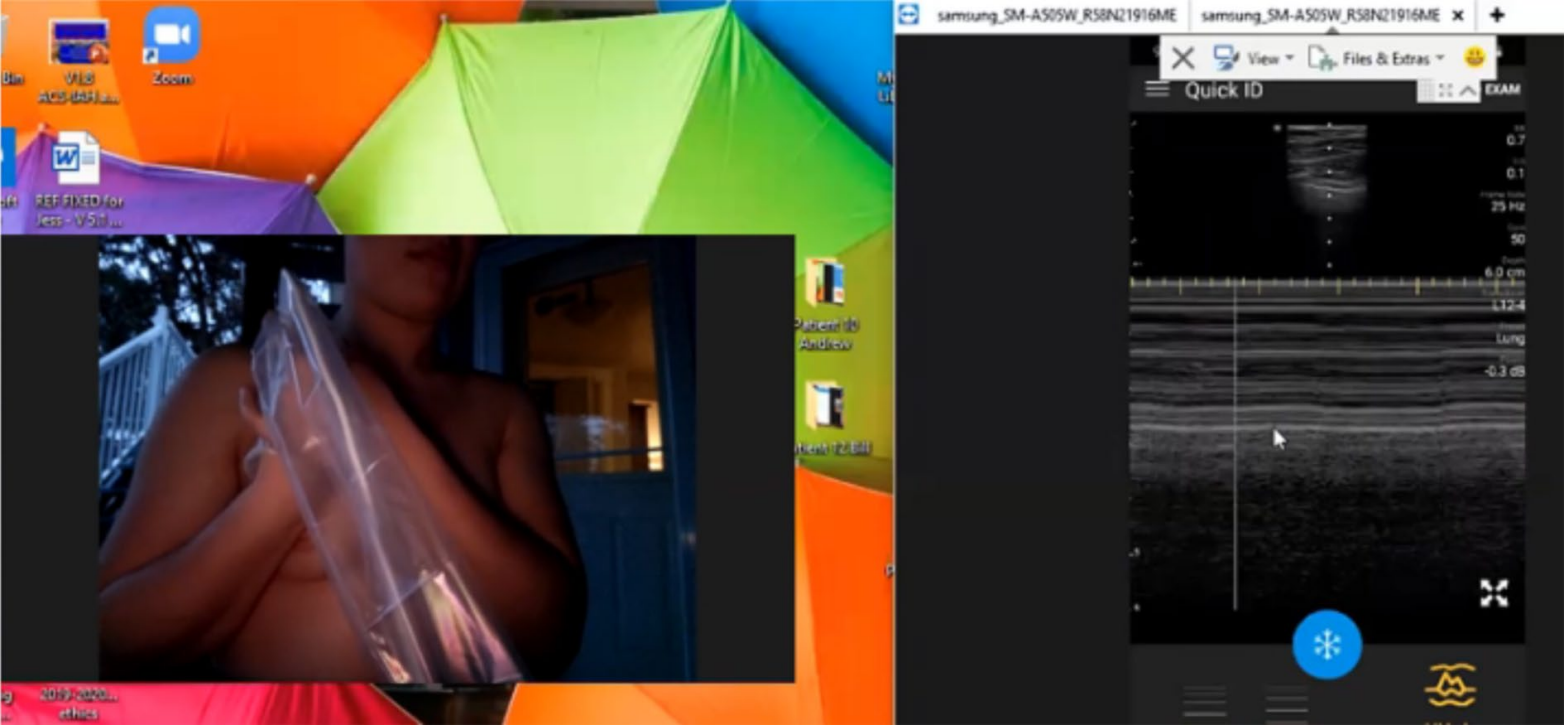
Baseline Self-Performed Lung Ultrasound Study of 31-year-old potentially exposed patient-spouse 6 months prior to patient COVID-19 Diagnosis. Screenshot of the Remote Mentors Computer Screen that shows the patient imaging herself to the left of the screen and the resultant ultrasound image showing the “seashore” sign to the right. As lung ultrasound is a dynamic real-time examination hard to capture in any single image, readers are strongly encouraged to view the accompanying supplemental videos of the entire examination online to gain a true appreciation of the technique
